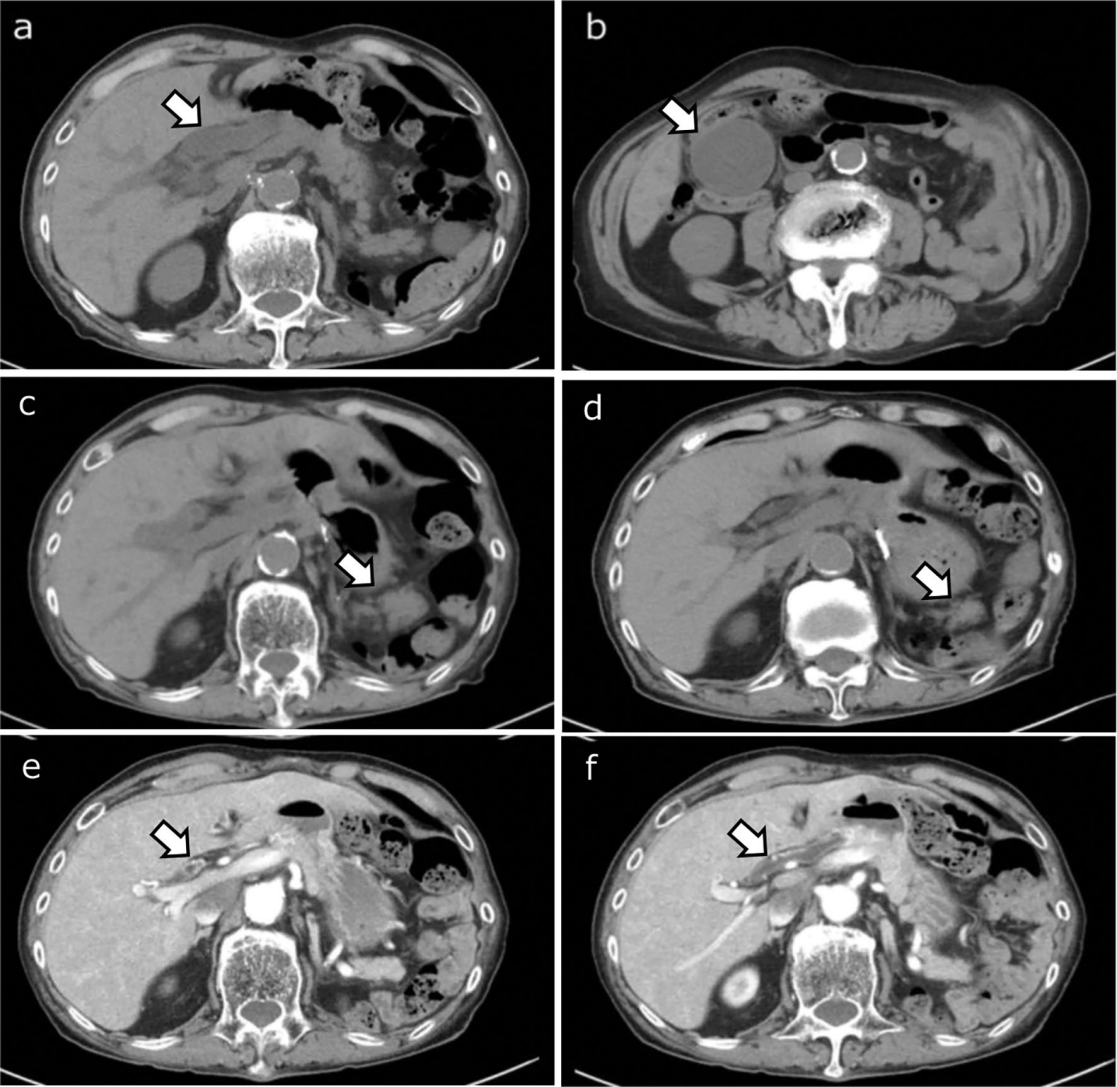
(a, b, and c): Non-contrast computed tomography (CT) at the time of the emergency department visit. (d): Non-contrast CT taken 3 months prior to the emergency department visit. (e and f): Early-phase contrast-enhanced CT (after antibiotic treatment). Mild dilatation of the common bile duct (a; arrow) and gallbladder enlargement (b; arrow) are noted. The pancreatic tail is also swollen compared to that of three months ago (c, d; arrows). Dynamic CT revealed heterogeneous enhancement in liver parenchyma (e) and circumferential thickening of the bile duct walls, extending from the hepatic hilum to the distal bile duct (e, f; arrows)
